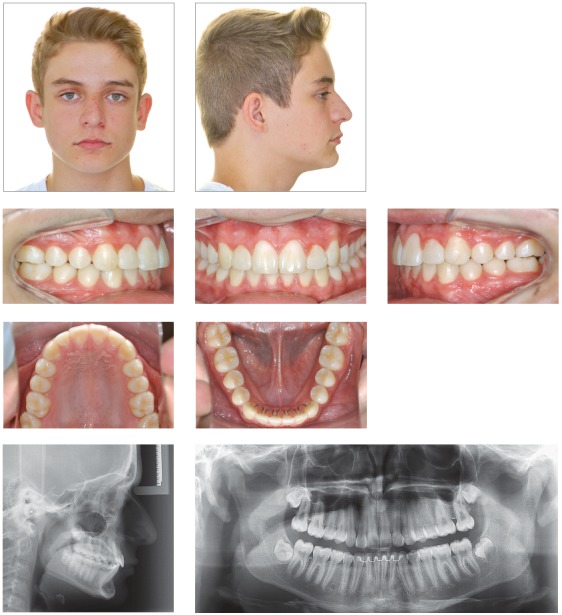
Final records: extra- and intraoral photographs, lateral cephalogram and panoramic radiograph.
